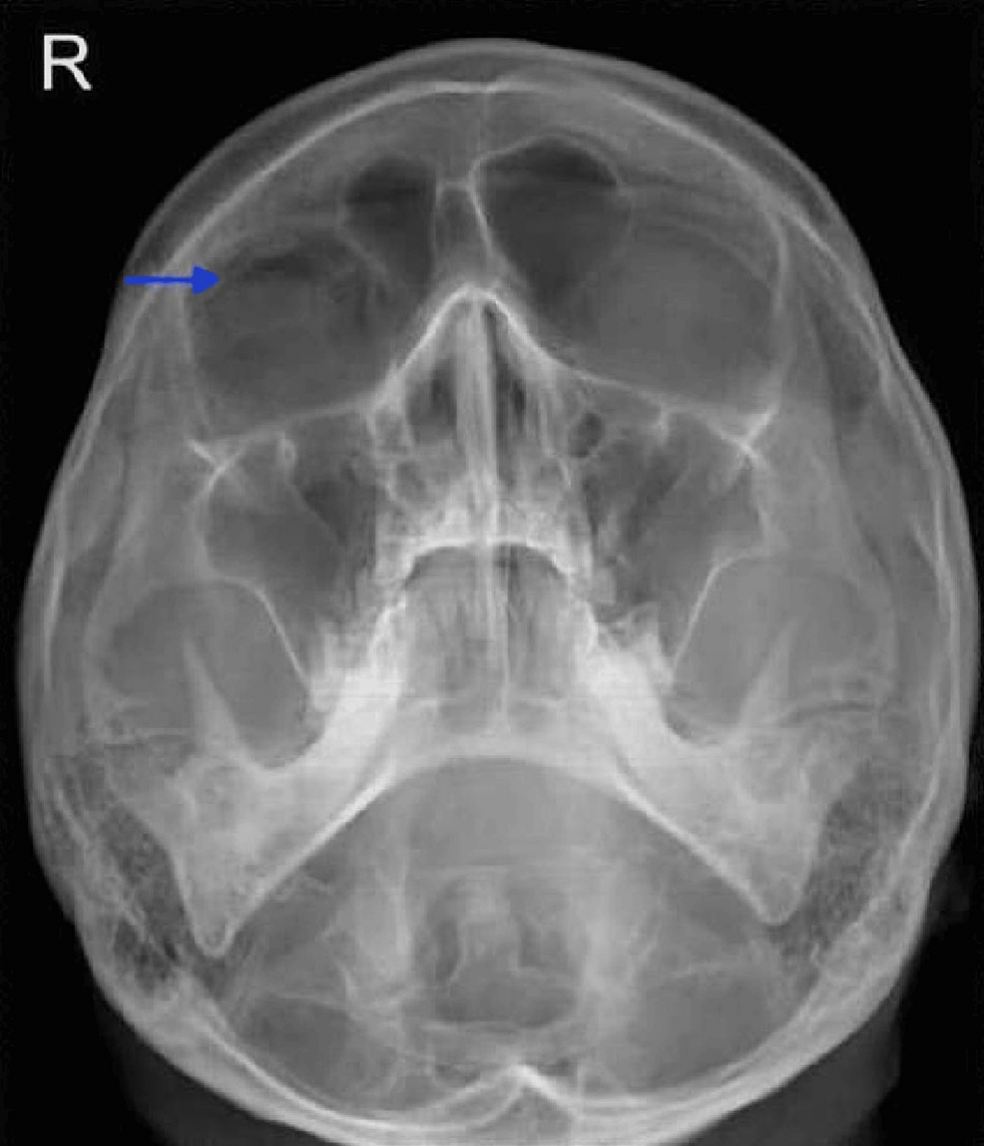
Plain skull X-ray occipitomental view (Waters’ view) showing a “black eyebrow” sign, as depicted by the blue arrow.
